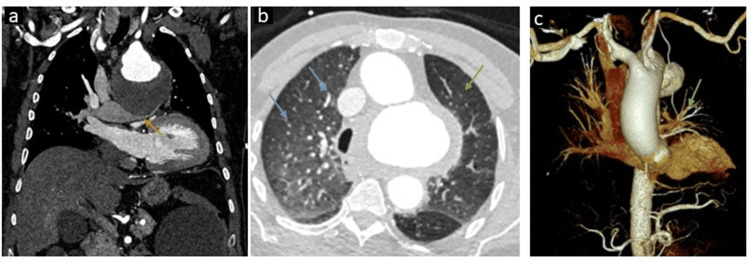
Computed tomography demonstrating the mass effect on the left main pulmonary artery with resultant pulmonary oligemia (A) Coronal image demonstrating severe compression of the left main pulmonary artery (yellow arrow) by the giant aortic arch aneurysm. (B) Axial image in lung window at the level of bilateral upper lobes showing a reduced caliber of the segmental and subsegmental pulmonary arteries, with resultant hyperlucent left lung parenchyma suggesting oligemia (green arrow). Note the normal-sized right upper lobe segmental and subsegmental pulmonary arteries (blue arrows). (C) 3D virtual rendering technique (VRT) image demonstrating a marked reduction in the size of the left pulmonary vasculature (green arrow) at the level of the hilum due to compression of the left main pulmonary artery.
